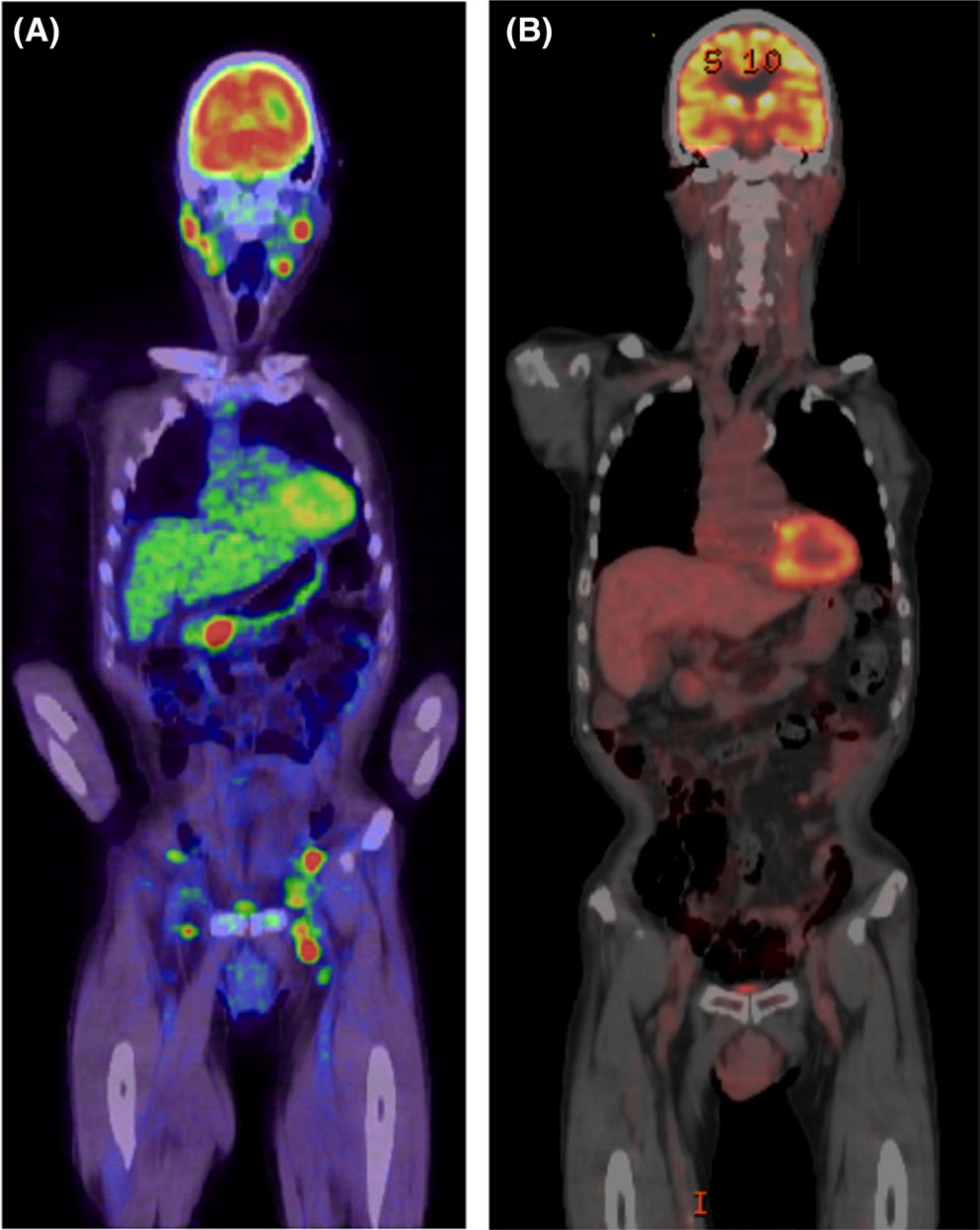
PET/CT images during the diagnosis of the high‐grade B‐cell lymphoma. A) Fused positron emission tomography showing increased fluorodeoxyglucose uptake at the bilateral cervical and also around the pancreas head and bilateral inguinal regions. B) The metabolic CR was maintained after 6 courses of EPOCH‐R therapy
